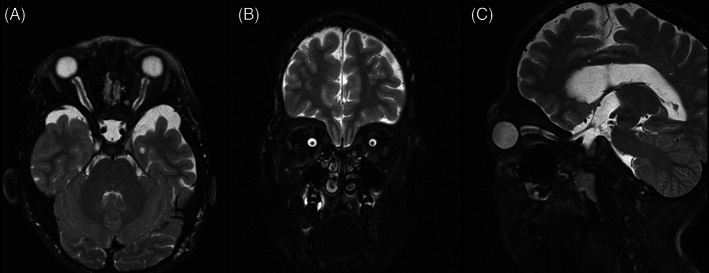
T2‐fat saturation MRI for patient 2 showing enlarged subarachnoid space with the “sausage” sign in sagittal section. (A) Axial section; (B) coronal section; (C) sagittal section
